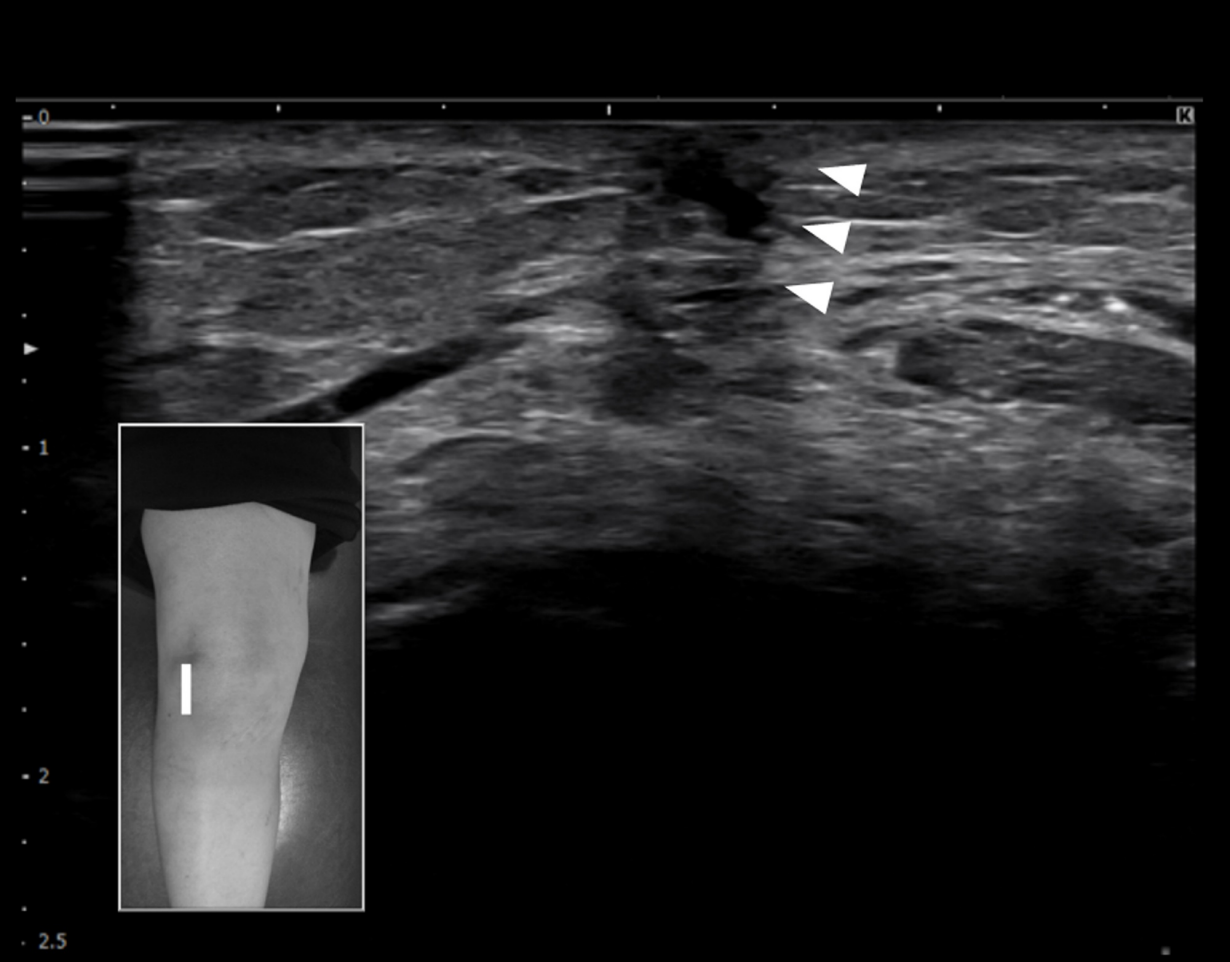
After ultrasound-guided hydrorelease The hypoechoic in the scar area (arrowhead) is attenuated and sliding is improved when the skin is manually slid. The surface of the skin is no longer pulled deep.
